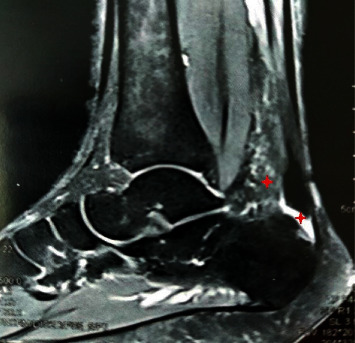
Magnetic resonance imaging T_2_ sequence, sagittal view showing an increased signal intensity of the retrocalcaneal bursa with marked fibrosis anterior to the Achilles tendon (red asterisks).
